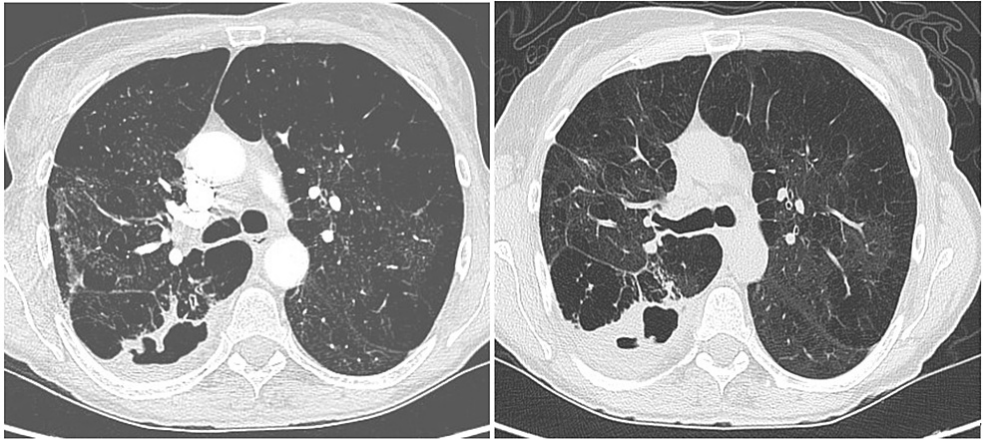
Computed tomography scan of the chest at the level of tracheal bifurcation showed cavitary lesion at the first visit (left) and second visit (right)
